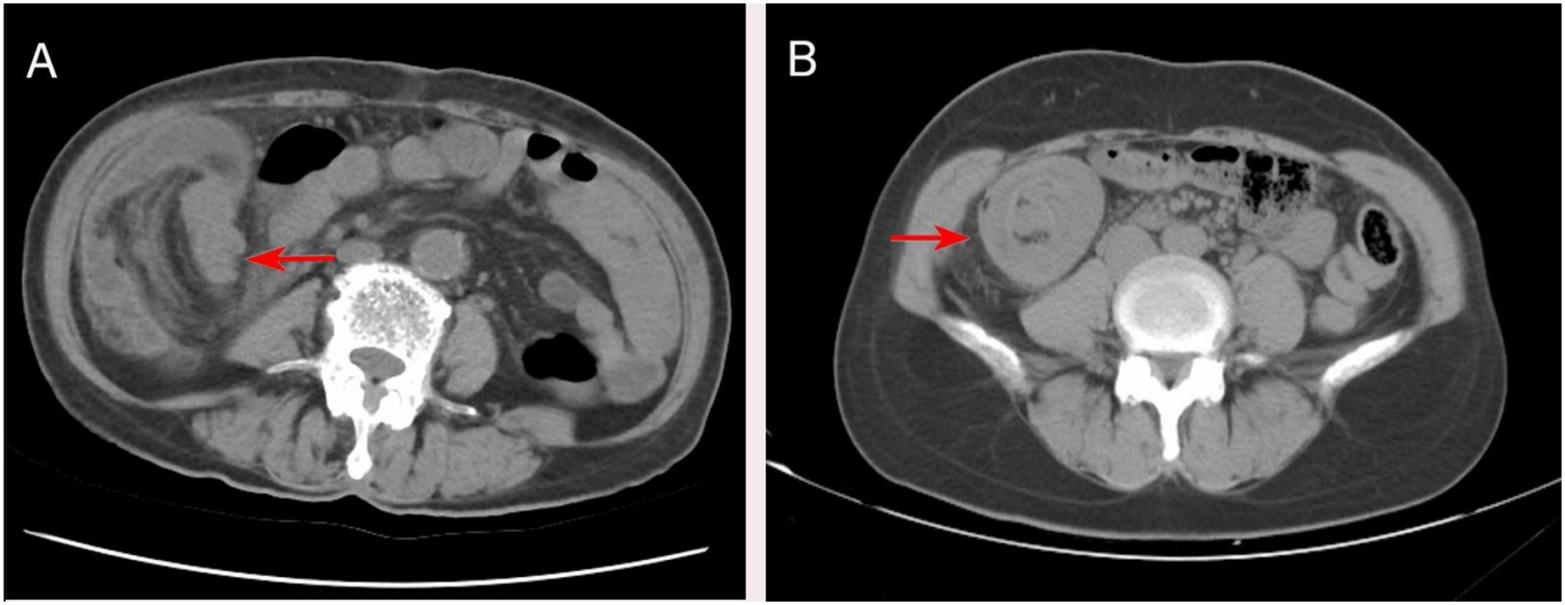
(A) CT at the level of the abdomen shows ileocecal intussusception (arrow). (B) CT at the level of the abdomen shows a round, target-shaped mass in the right abdomen (arrow).
